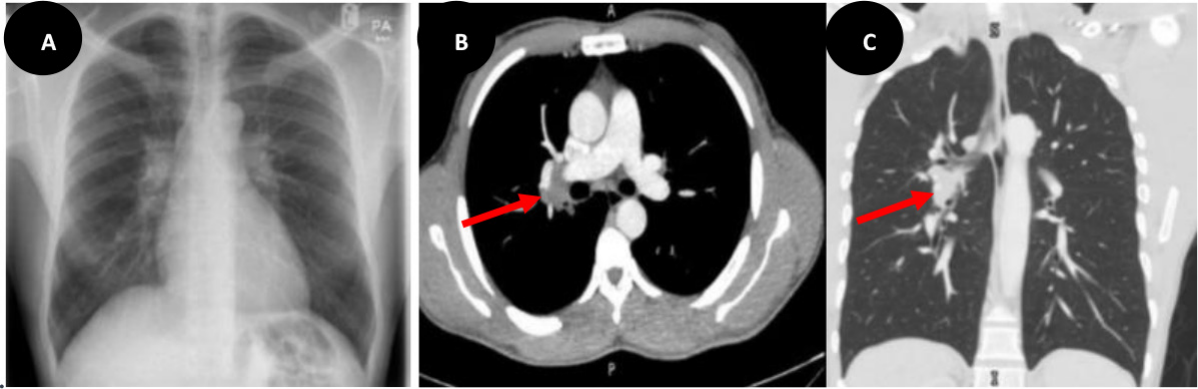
A chest radiograph demonstrates a prominent hilar regions (A). An axial and coronal CT thorax views showing single enlarged right hilar lymph node with otherwise clear both lungs fields (B & C).
